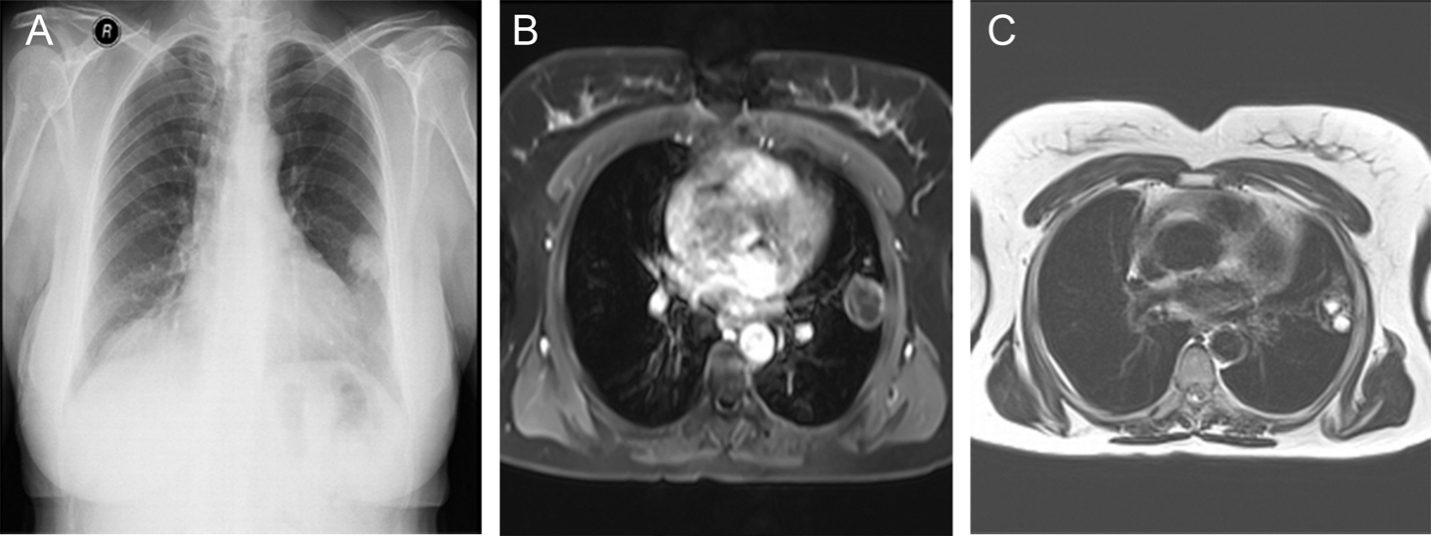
Posteroanterior chest x-ray (A), fat-suppressed contrast-enhanced T1-weighted magnetic resonance image (MRI) (B), and T2-weighted MRI (C) showing the left pulmonary hydatid cyst.
